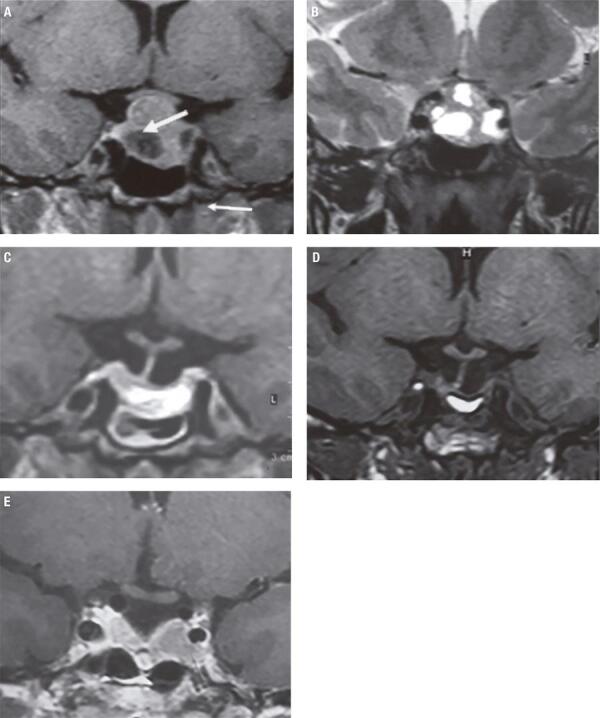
Case 2 – Pre-surgery pituitary MRI (A-B): Coronal view of a heterogeneous lesion with solid and cystic areas. T1 after gadolinium administration (A) and T2-weighted images (B) showed left cavernous sinus and suprasellar extension with compression of the optic chiasm. Post-surgery MRI (C-E): Coronal T1-weighted image showed partial empty sella and spontaneously hyperintense filling material. No visible tumor remnant (C) MRI performed after 6 months of a non-complicated pregnancy; the lesion remained stable (D). MRI six years after pregnancy. Contrast-enhanced coronal T1-weighted image shows a small tumor recurrence close to the left cavernous sinus (E).
